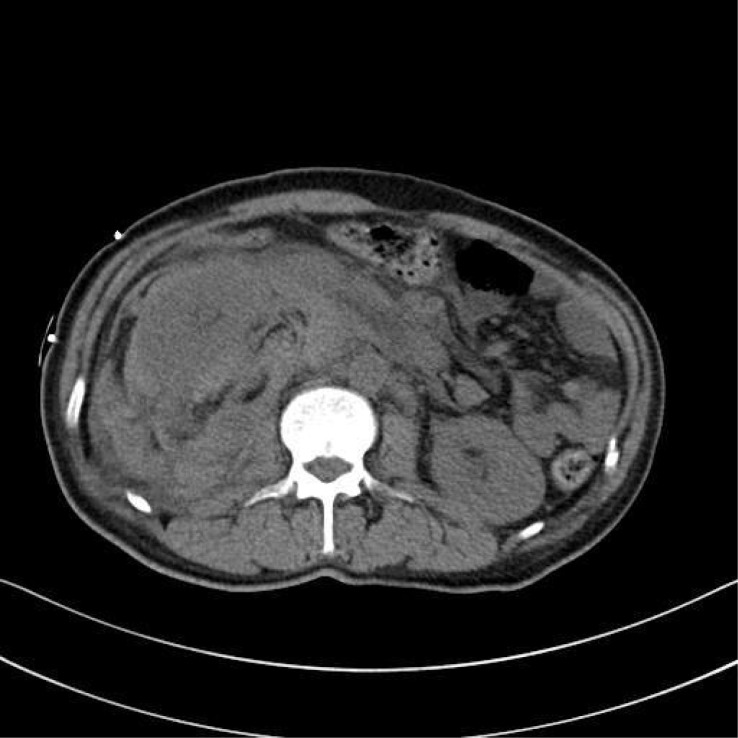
CT cross-sectional images revealing a perirenal hematoma on the right kidney.
